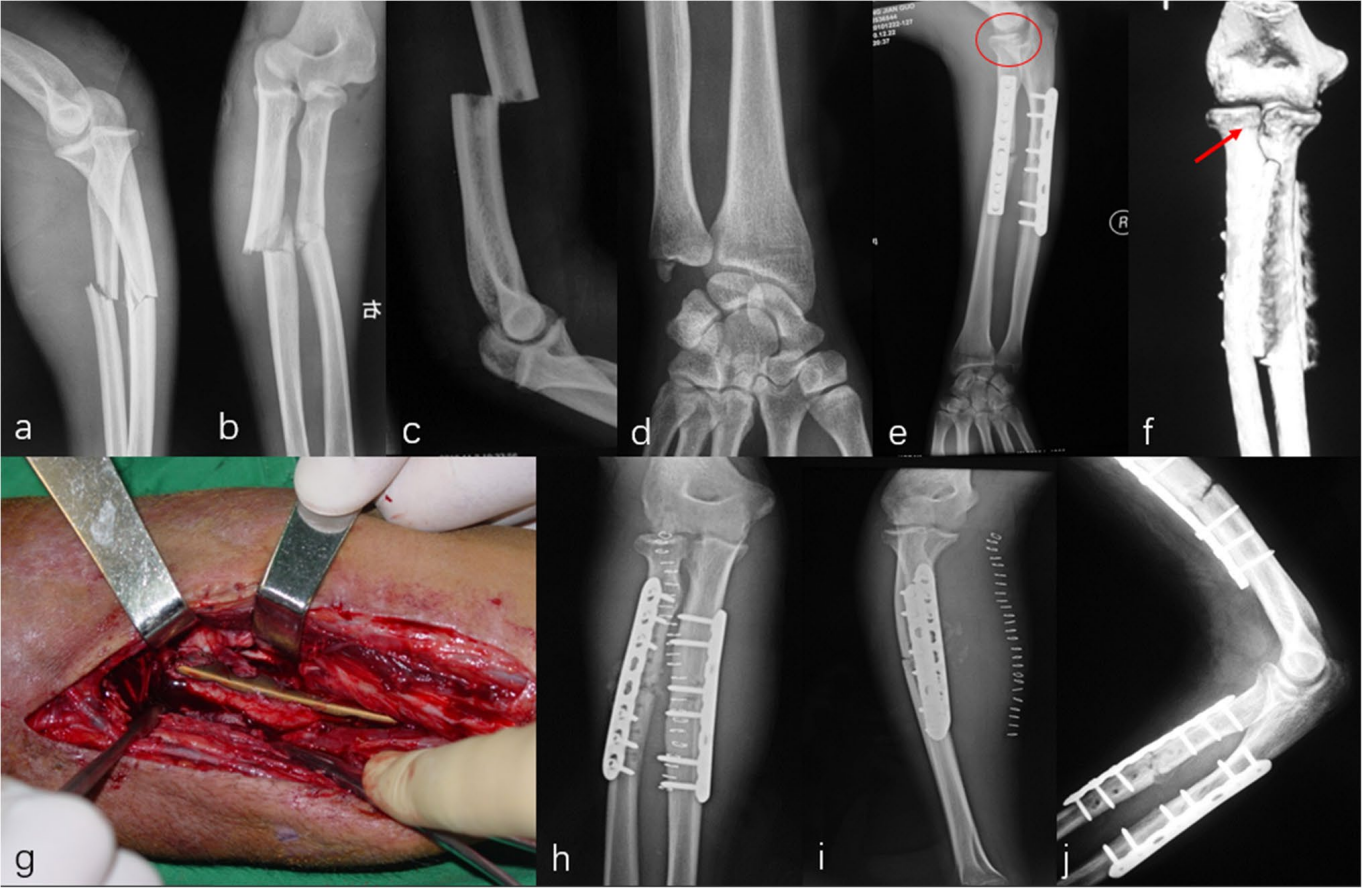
Post-operative radiographs of the right elbow revealed a DDE and a multiple fracture (a–d). However, this patient sustained an elbow malrotation (arrow and circle), which failed to restore the anatomical radial bow (e–f). Eight weeks later, a secondary operation consisting of radius shortening and release of an elbow contracture was required to restore motion (g–j)
